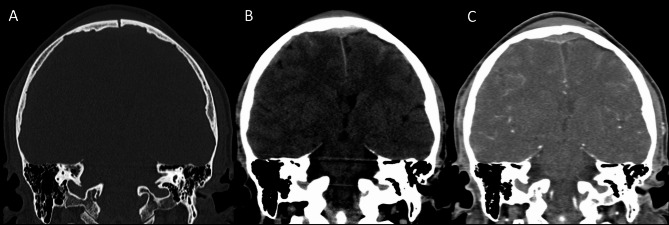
Blunt trauma in a patient in their twenties. Non-enhanced brain CT shows a post-traumatic fracture of the frontal bone (A), with evidence of an extra-axial blood collection, consistent with midline epidural hematoma of the cranial vault (B). CTV (C) demonstrates lack of opacification of the superior sagittal sinus, resulting from compression by the epidural hematoma
